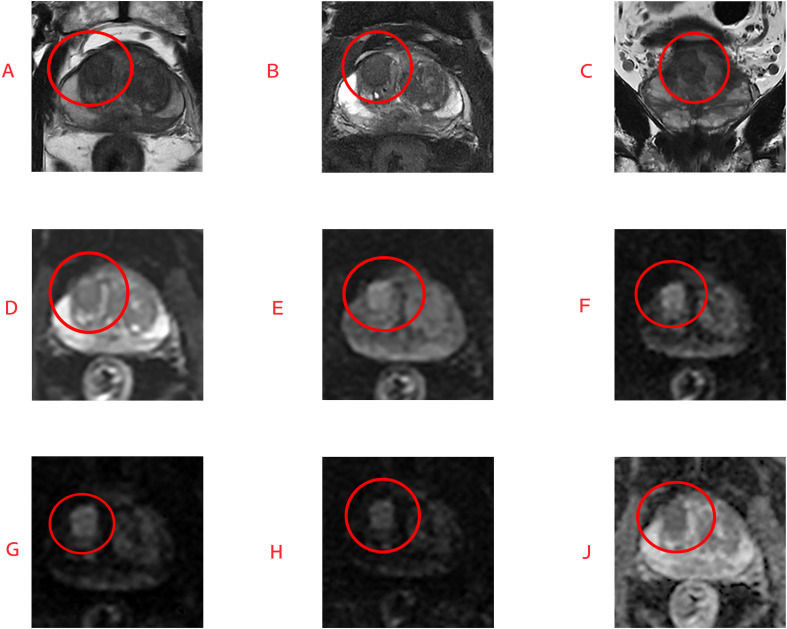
The patient is 65 years old. MRI analysis revealed a lesion scoring three on T2-weighted images (A–C) and five on DWI, diffusion-weighted imaging sequences (D–J). Subsequent biopsy pathology results showed a GS, Gleason score of 8, confirming the superior diagnostic value of DWI scoring in prostate cancer assessment.
